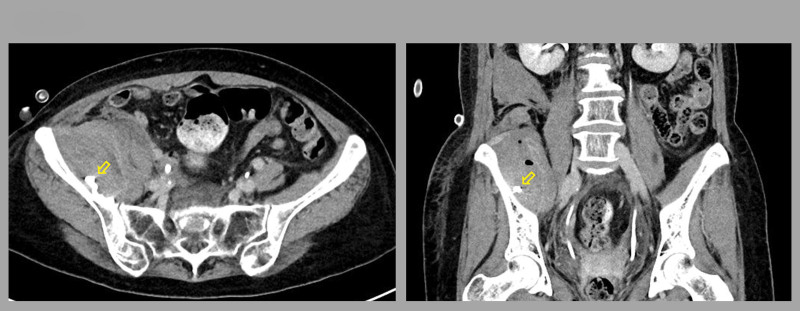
Contrast-enhanced abdominal computed tomography image of post-embolization state (yellow arrow, left: transverse view, right: coronal view).
